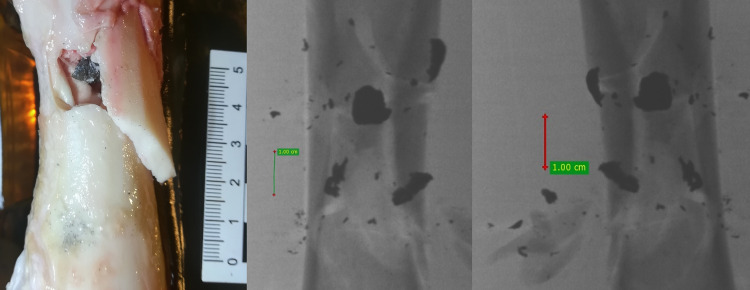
H&N Baracuda Hunter pellet cal 6.35 mm, from the left: entry wound damage, A-P X-ray, lateral X-ray. Shot to the posterior surface of the femoral shaft.
